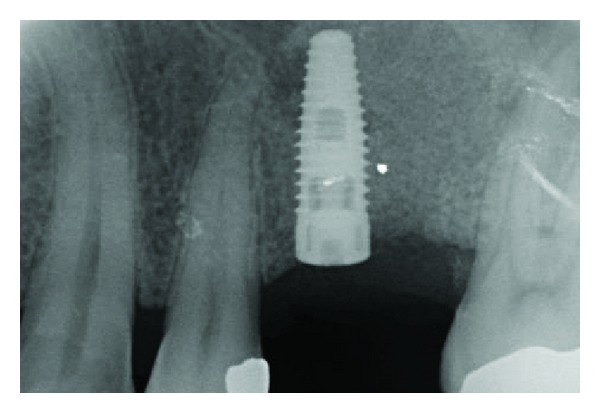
X-ray control after 3 months.
